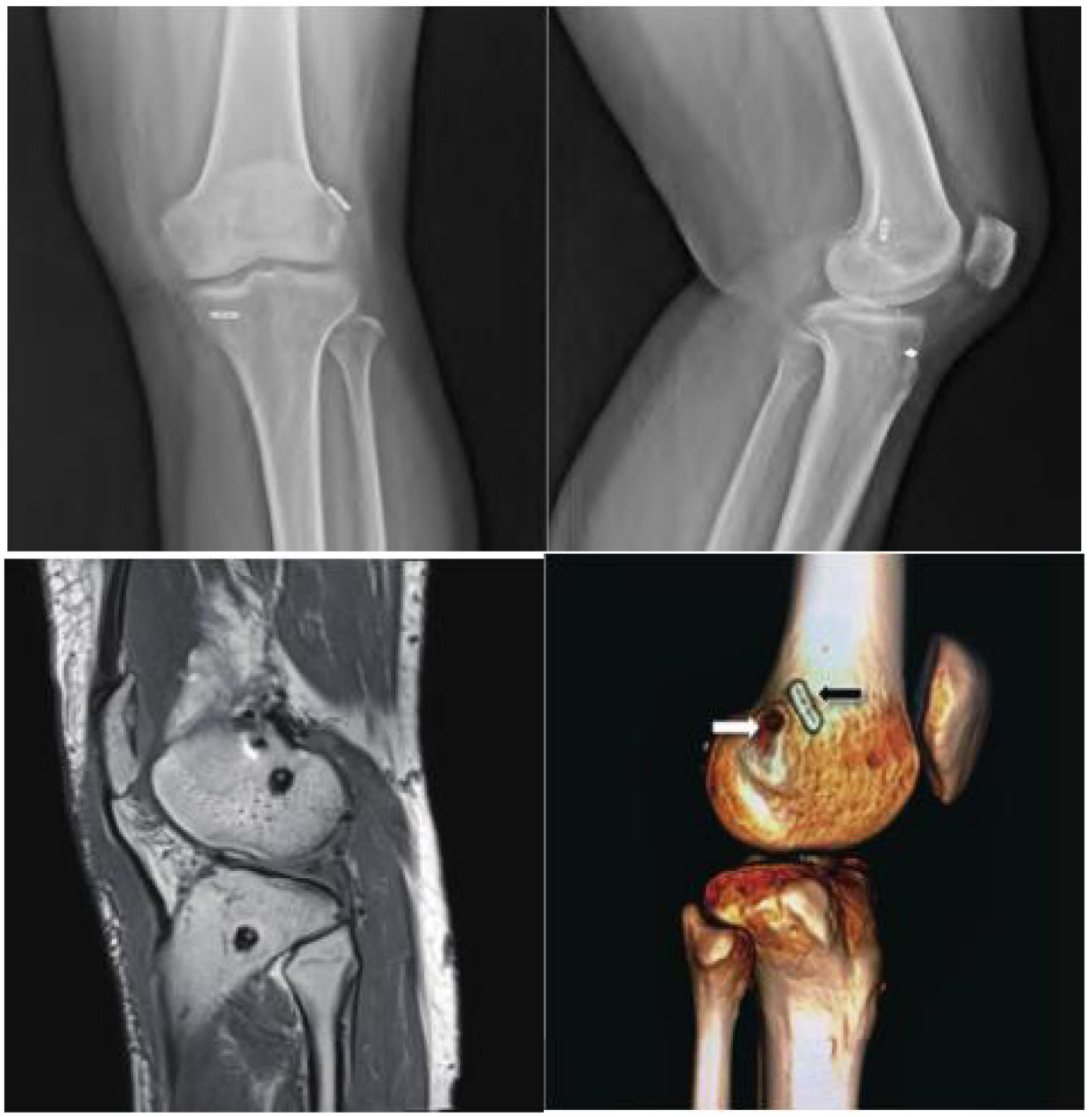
X-ray, MR, and CT images after total internal single beam reconstruction.
